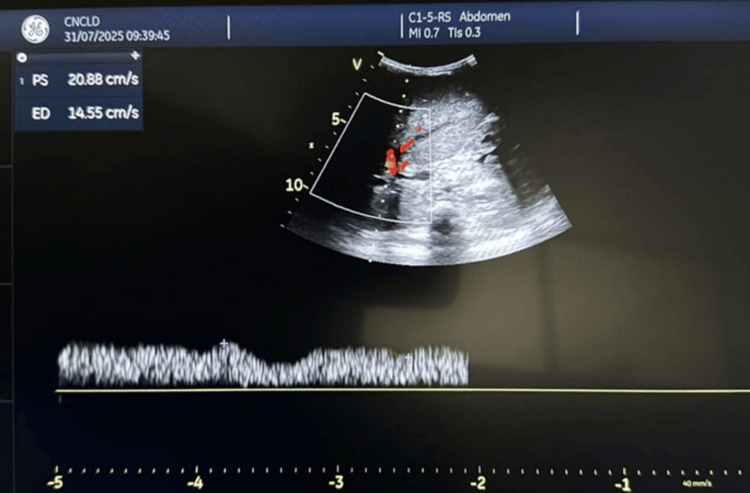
Postoperative Doppler ultrasonography confirming adequate portal-venous flow (peak velocity ≈ 25 cm/s)
